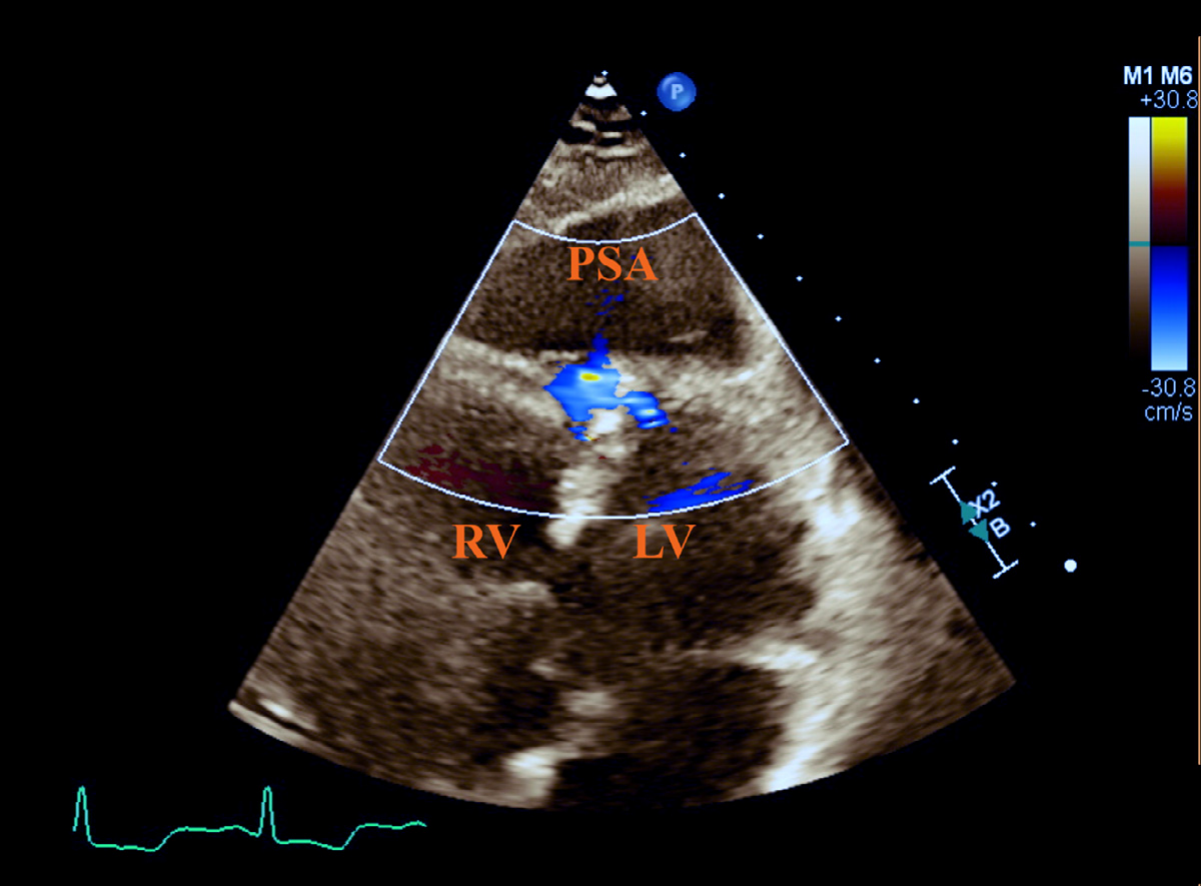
Transthoracic apical 4-chamber echocardiogram with color Doppler flow across the anterior right and left ventricular walls, centering over the interventricular septum and emptying into the ventricular pseudoaneurysm (PSA).
